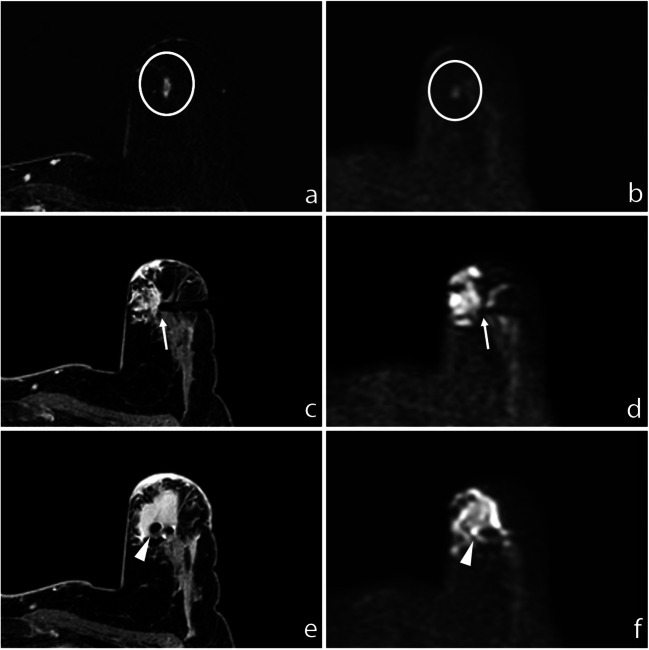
a Subtracted DCE 3D-THRIVE showing a mass lesion in the upper inner quadrant of the left breast of a 48-year-old woman (circle). b DWI (*b* = 1300 s/mm^2^) showing hyperintensity of the enhancing lesion (circle). c T1-WI FS GRE showing the proper position of the biopsy device (arrow); the needle is well recognizable at (d) DWI (*b* = 1300 s/mm^2^, arrow). e T1-WI FS GRE showing the correct deployment of the site marker that is also recognizable at (f) final DWI (*b* = 1300 s/mm^2^, arrowhead)
